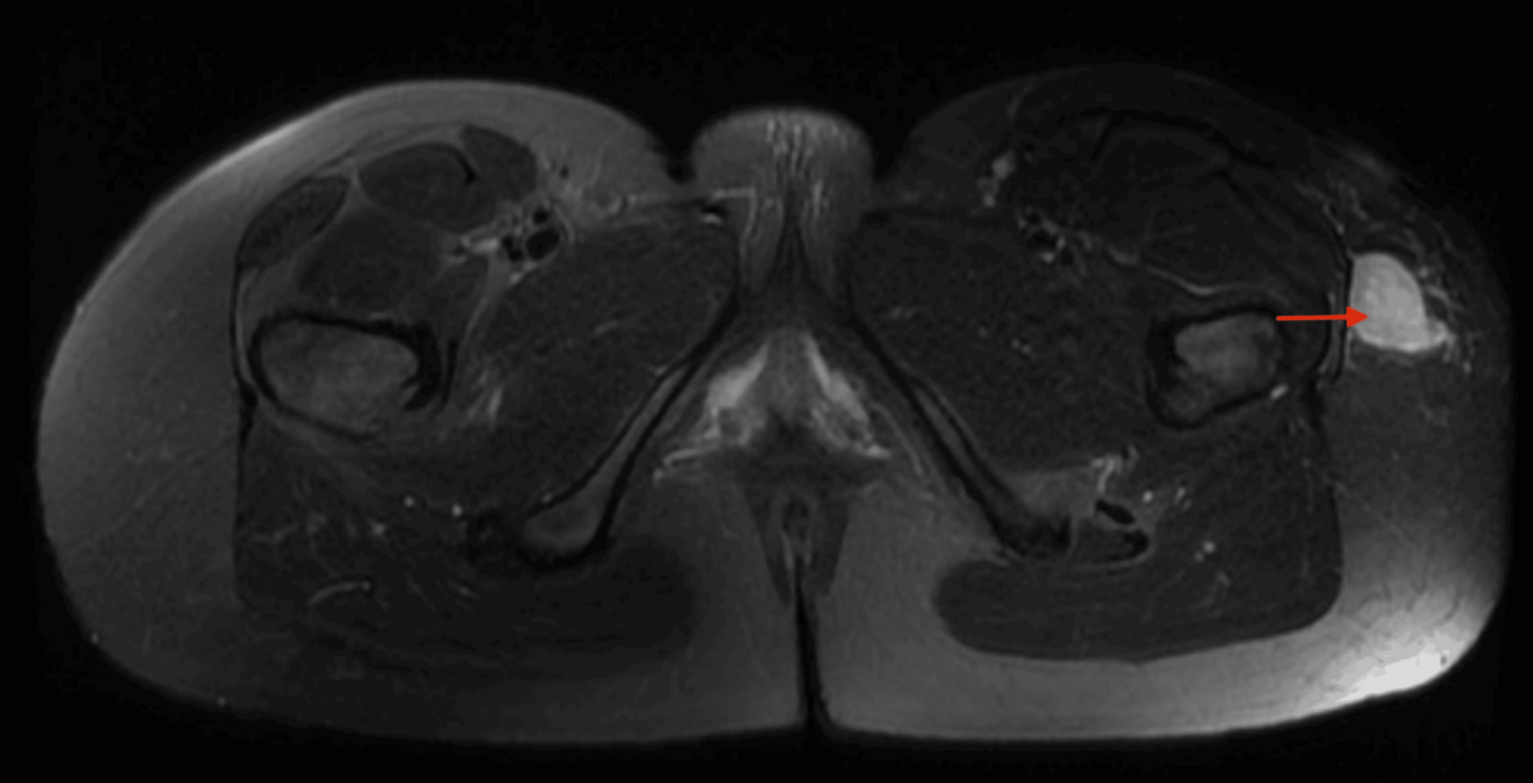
Axial T2-weighted fat-suppressed pelvic MRI shows a well-circumscribed, heterogeneously hyperintense superficial nodule along the lateral aspect of the left hip, adjacent to the tensor fasciae latae (red arrow). The surrounding fat planes remain preserved, with no deep fascial breach or extension into the underlying muscle.
